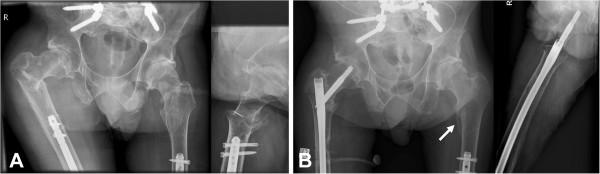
Plain radiographs taken 10 weeks after the final spinal surgery showing intertrochanteric right neck of femur fracture distal to the iliac fixation and subsequent intramedullary fixation. (A) Radiographs taken 10 weeks after the final spinal surgery showing intertrochanteric right neck of femur fracture distal to the iliac fixation. (B) Radiographs taken 6 weeks post-intramedullary fixation showing stable fixation of the right femoral fracture. A stress response with periosteal new bone formation in the left subtrochanteric region is apparent (arrow).
